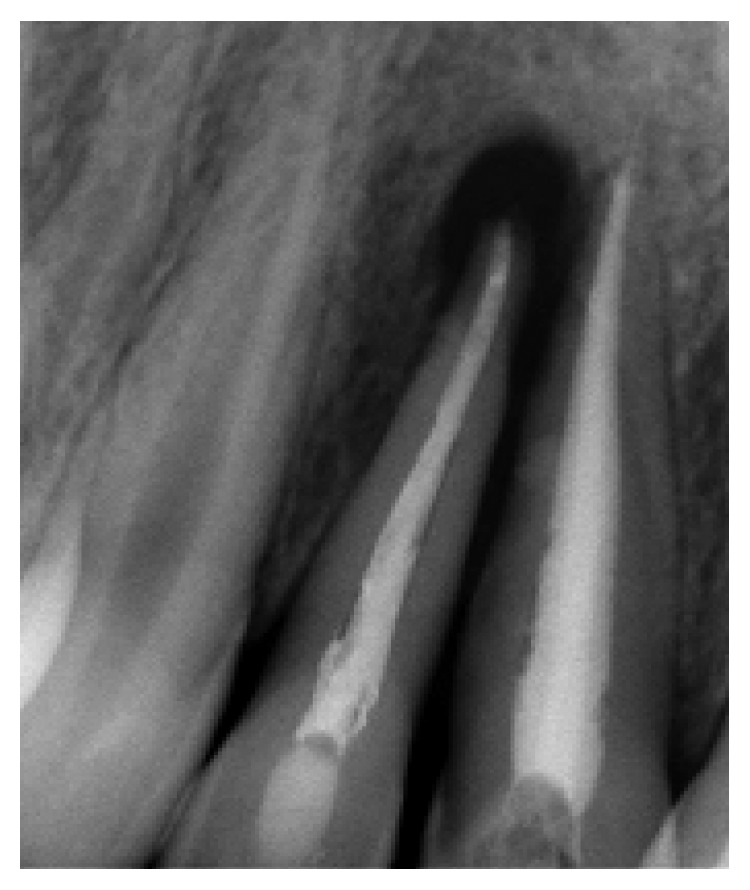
Radiograph of control after 1 month.
